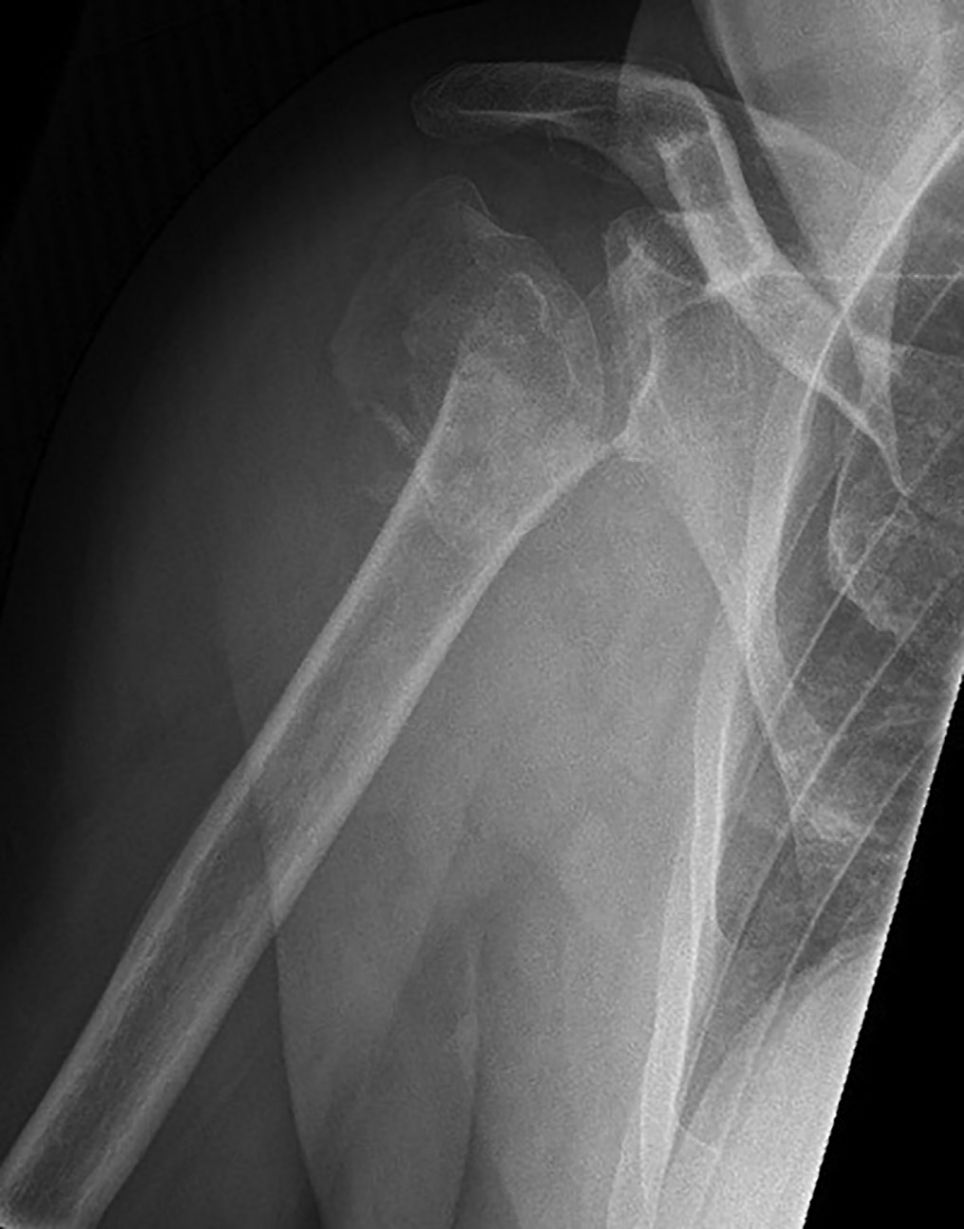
Radiograph from our emergency department displaying a three–four‐part fracture. The humeral shaft is medialized. The dent in the glenoid is believed to be caused by trauma. There is a potential head split component because of two overlapping circular components.
